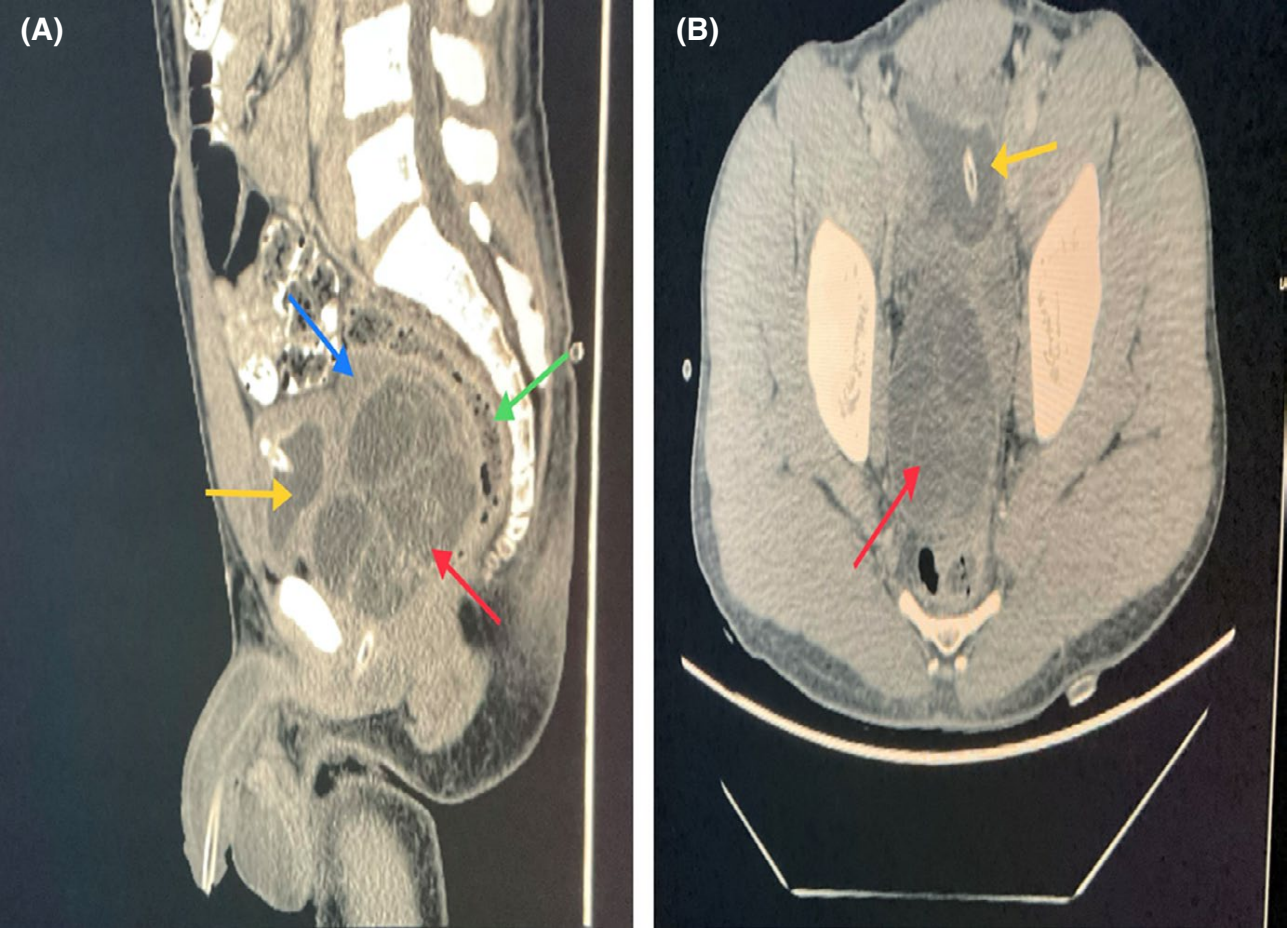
(A) Preoperative contrast‐enhanced CT scan with sagittal cuts, showing cystic lesion with enhancing septations compressing the rectum posteriorly (green arrow) and the urinary bladder anteriorly (yellow arrow) which appears under filled with Foley's catheter in situ. The seminal vesicle (blue arrow) appears compressed and pushed superiorly. (B) Preoperative contrast‐enhanced CT axial cuts, showing cystic lesion with enhancing septations (red arrow) compressing rectum posteriorly and urinary bladder anteriorly (yellow arrow) which appears under filled with Foley's catheter in situ
